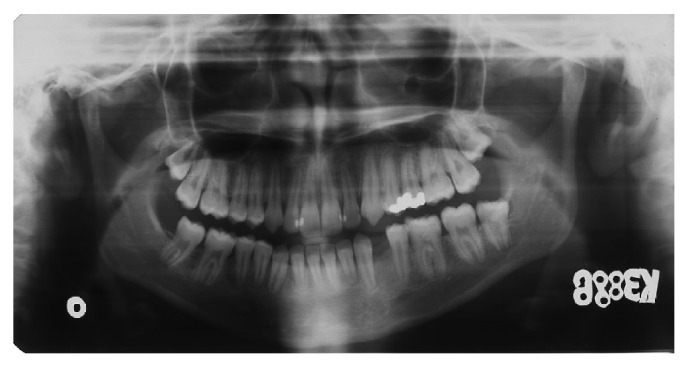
Posttreatment orthopantomograph.
